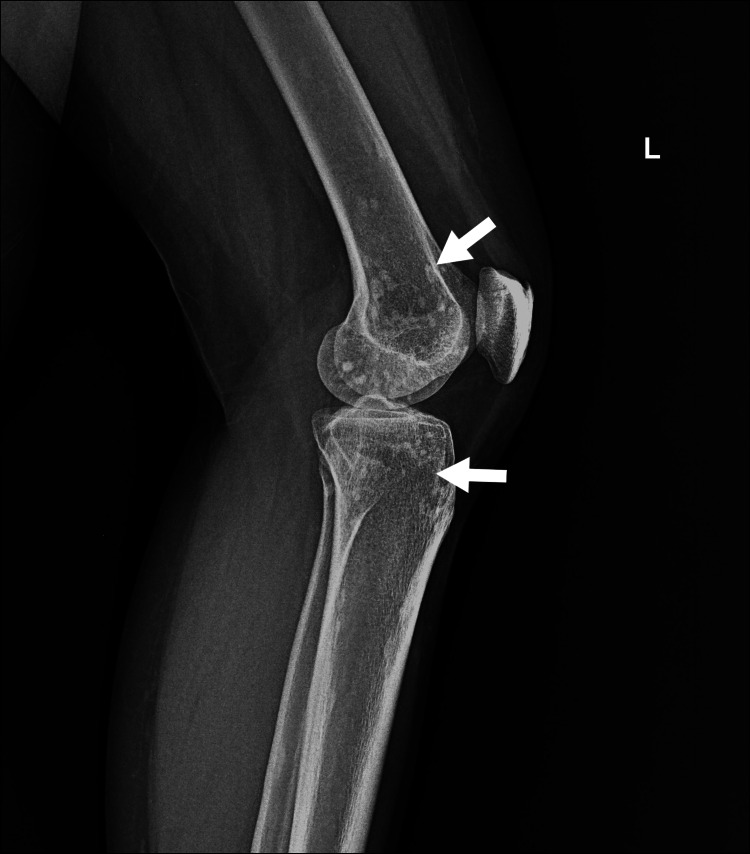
X-ray lateral view of the left knee joint White arrows show the multiple bony lesions in both the femur and tibia, which are symmetrical on the other side. L: left lower limb
